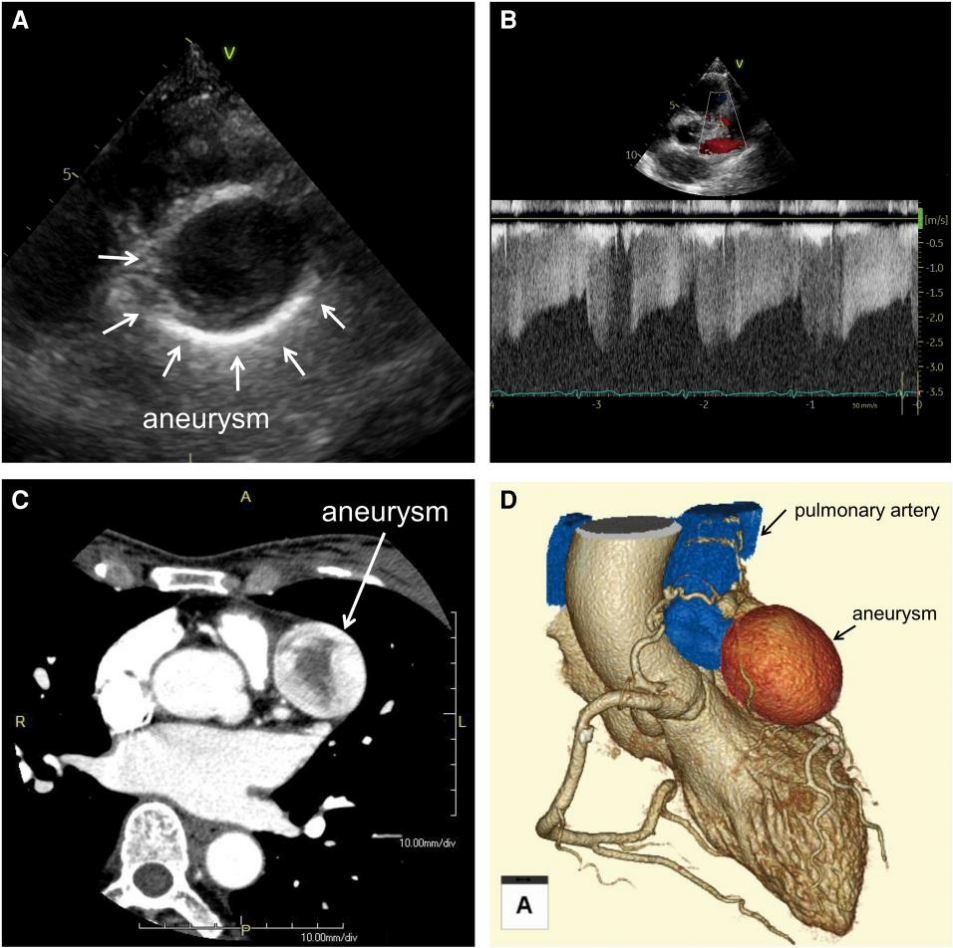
(*A* and *B*) Transthoracic echocardiography images and (*C* and *D*) contrast-enhanced computed tomography images at presentation.
